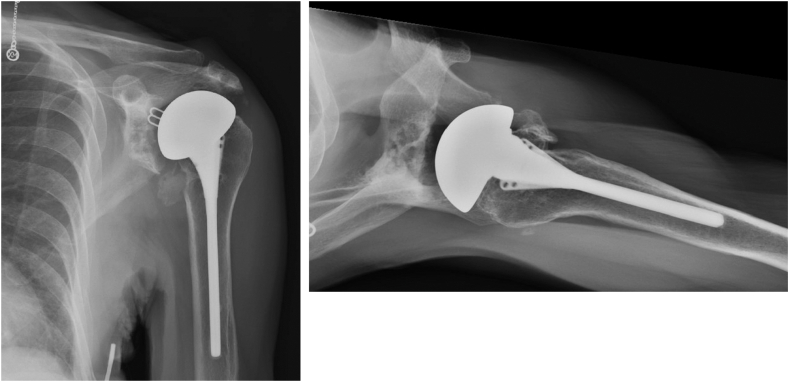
X-ray Grashey and axillary lateral view demonstrating no obvious fracture line in the shoulder.
